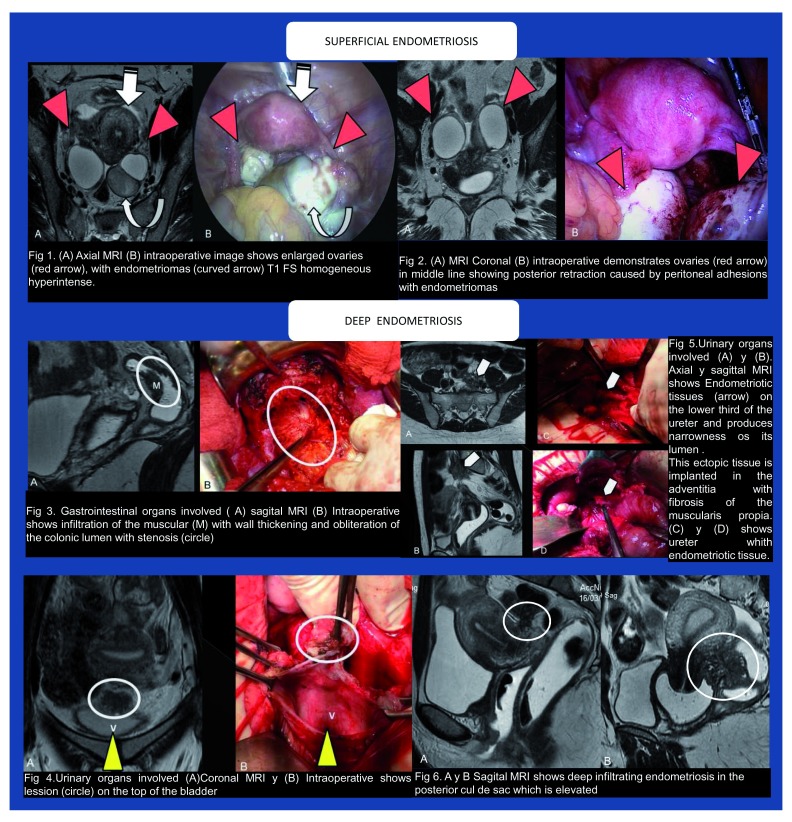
High-resolution magnetic resonance imaging and laparoscopic findings.
